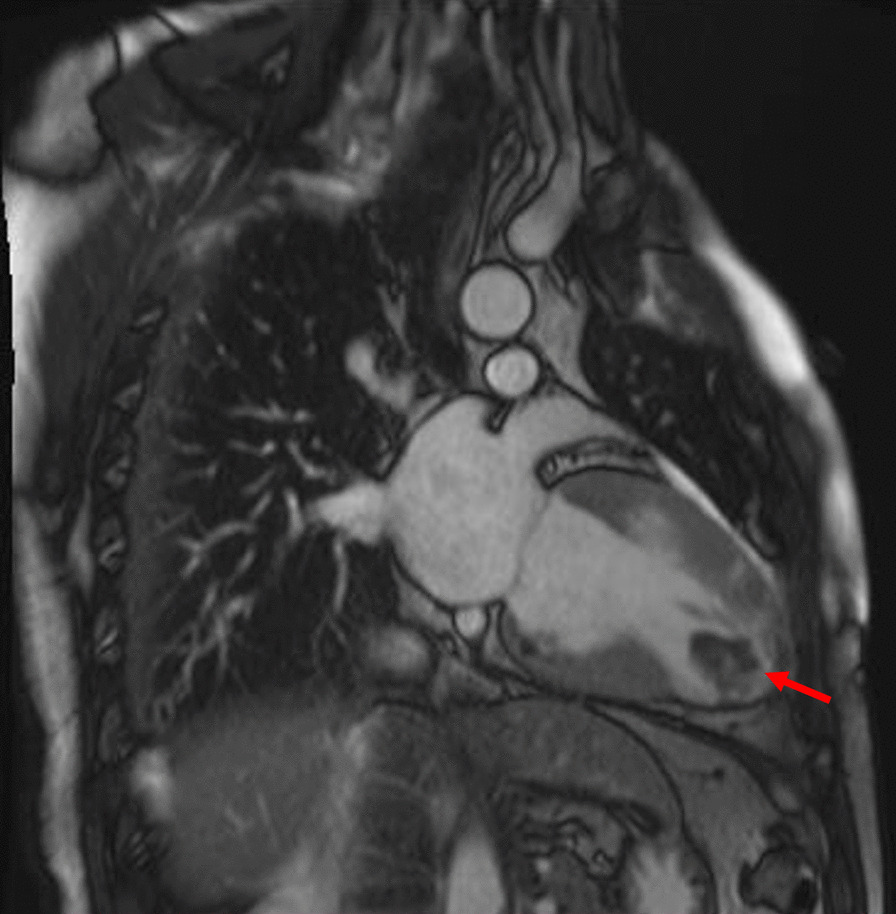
Presence of LV thrombus from magnetic resonance imaging study (arrow)
